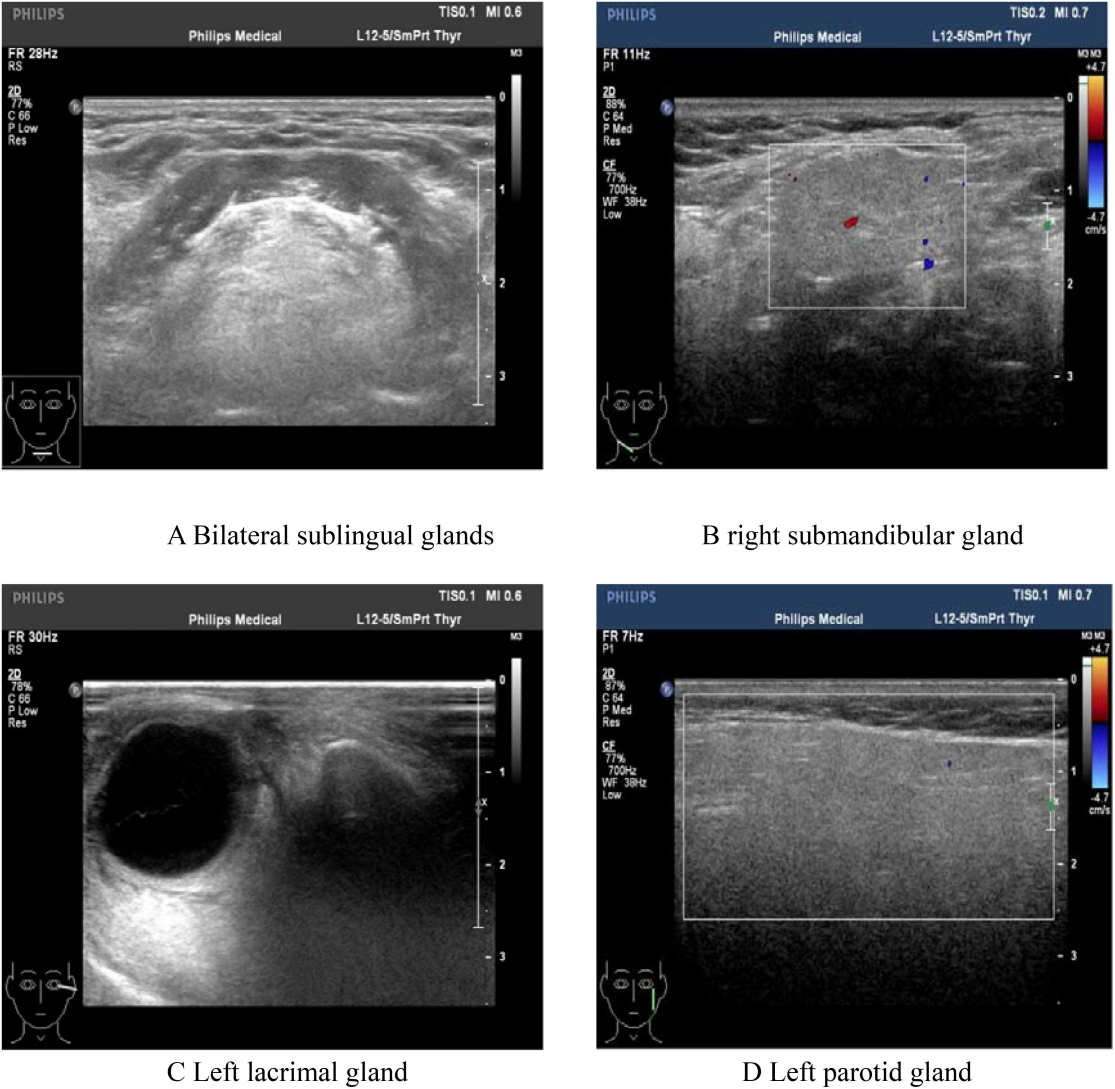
Color Doppler ultrasonography showed: heterogeneous changes in the bilateral lacrimal glands and submandibular glands. (A) Bilateral sublingual glands; (B) Right submandibular gland; (C) Left lacrimal gland. (D) Left parotid gland.
